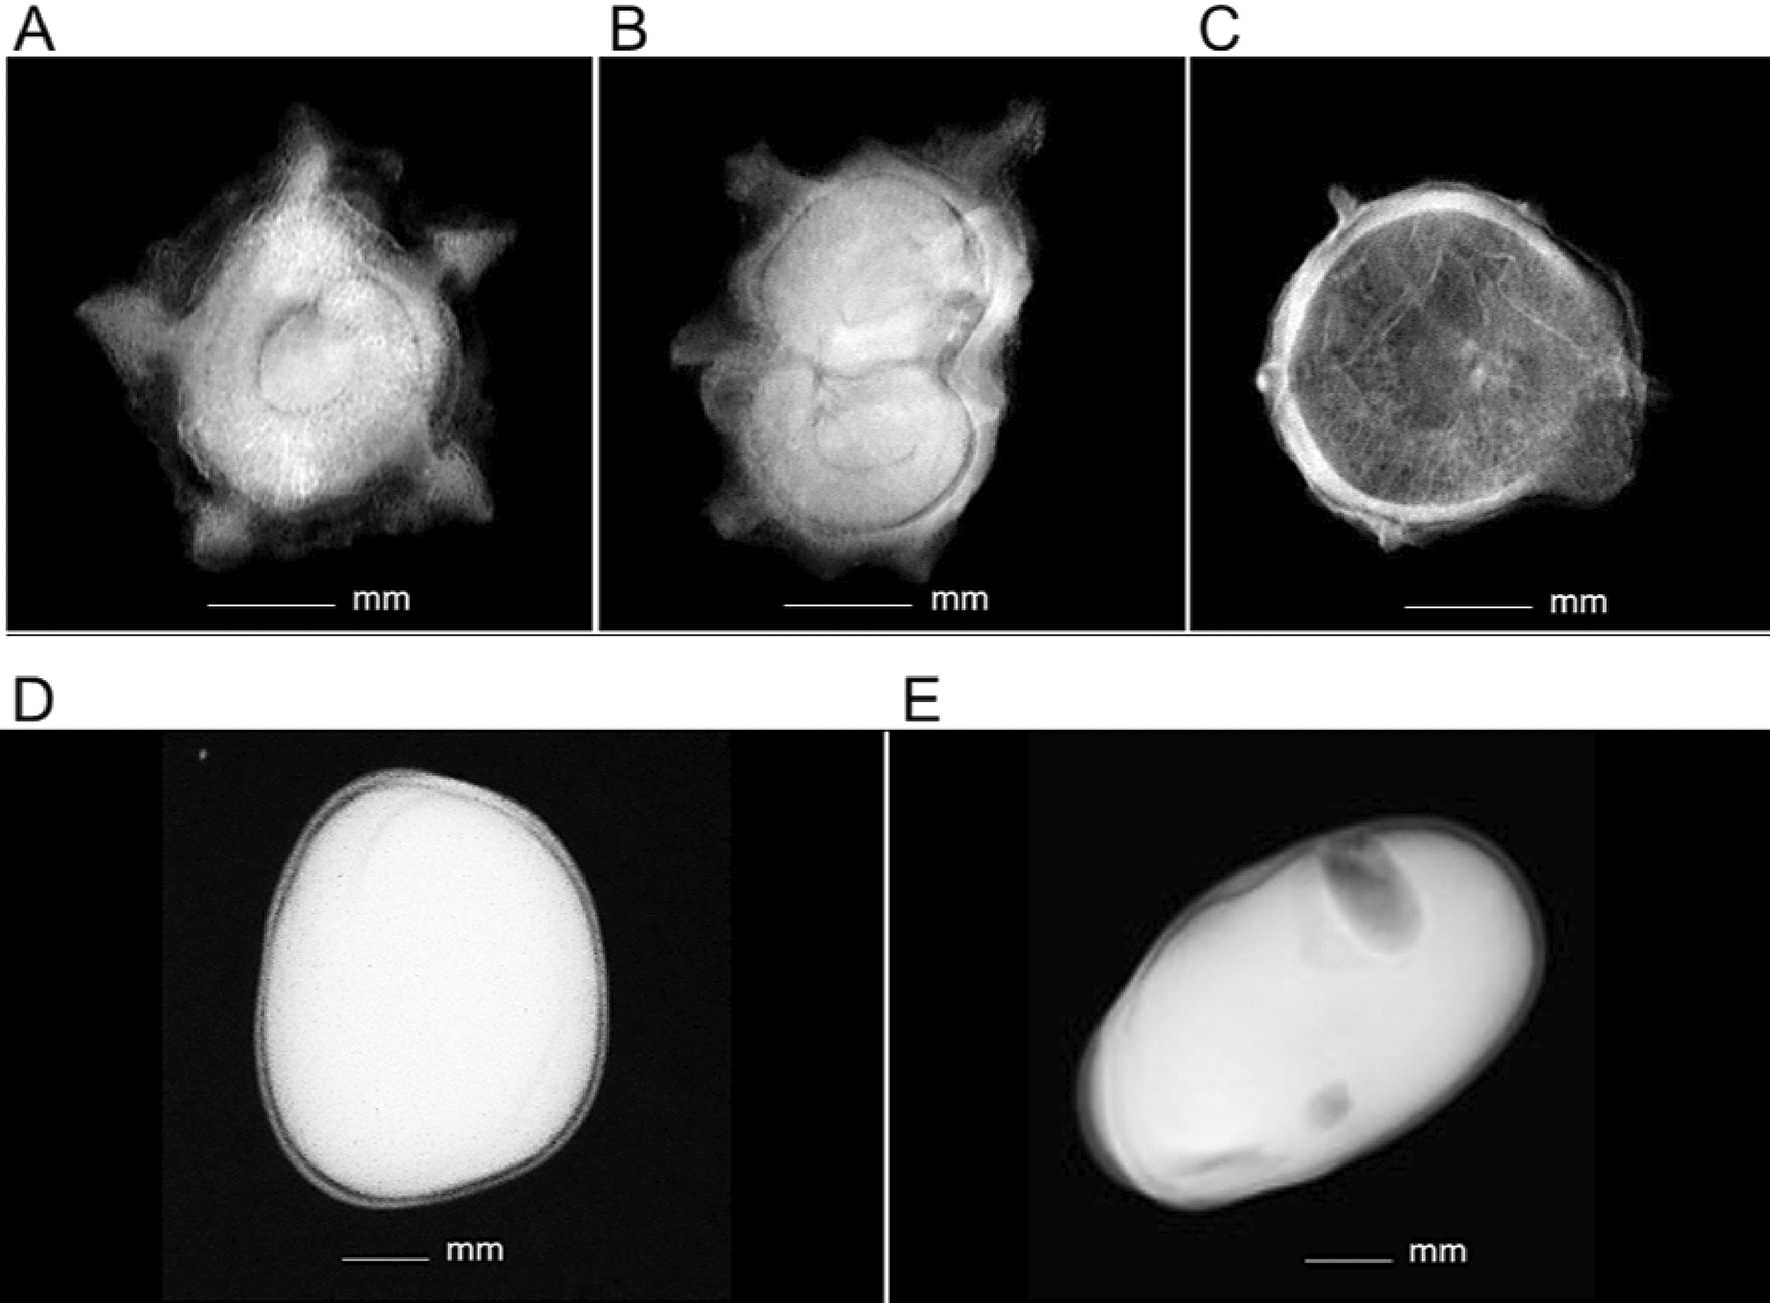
X-ray images of the classes used in this study. Three sugar beet classes based on the internal structure; (A) Monogerm, (B) Multigerm, (C) Empty, and two classes of faba bean seeds based on the physical quality; (D) Undamaged, (E) Damaged
